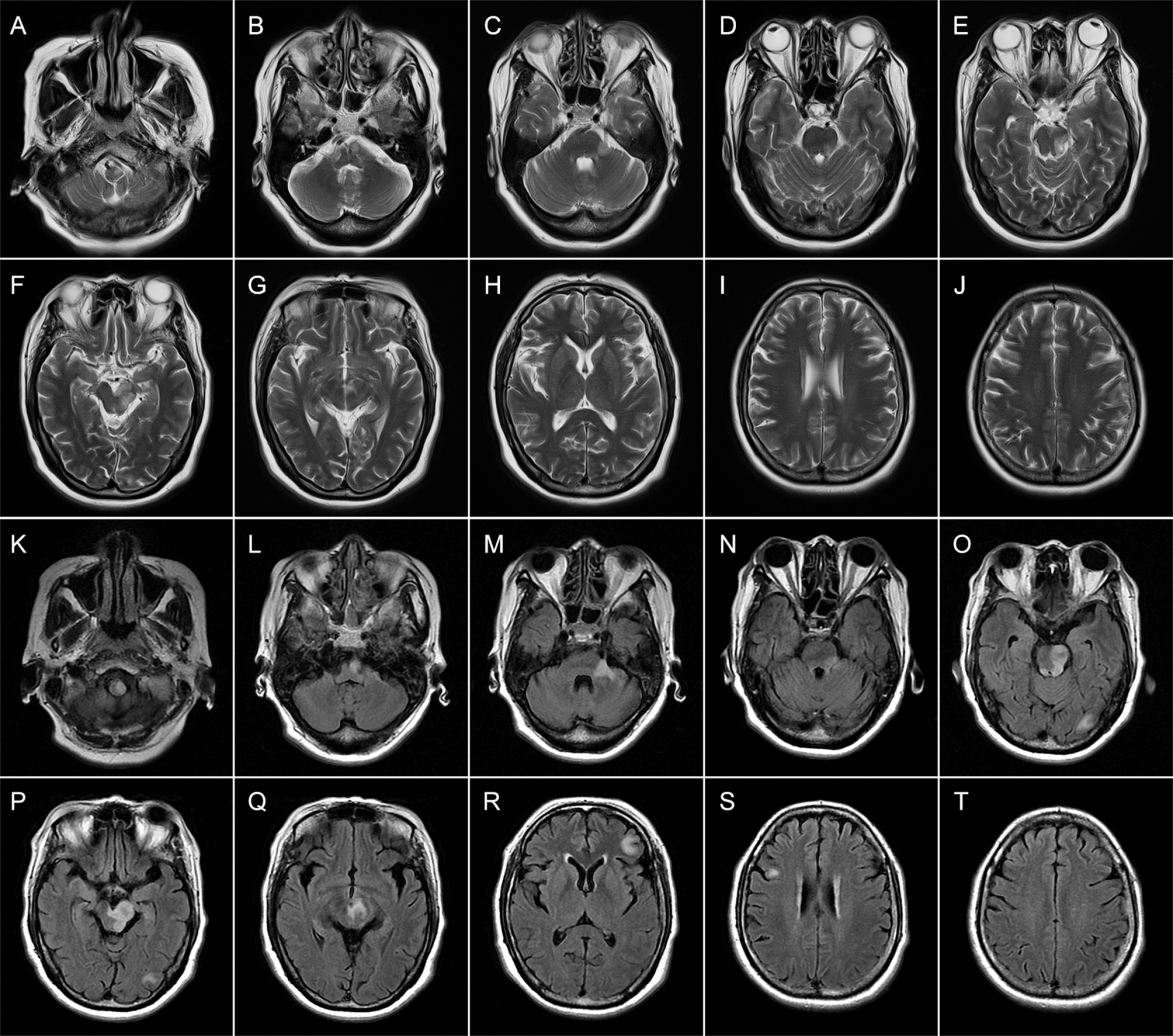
Brain magnetic resonance imaging (MRI) of patient 2 performed during acute attack. Axial T2-weighted (A–J) images obtained 4 days after onset show multiple patchy hyperintense lesions in the left brainstem (medulla, brachium pontis, and midbrain) (A–G) and juxtacortical white matters of the right frontal lobe (I). Axial FLAIR (K–T) images obtained 15 days after onset reveal expanding lesions in the brainstem (K–Q). Meanwhile, new patchy or spotty lesions are confirmed in the left occipital lobe (O, P) as well as juxtacortical and subcortical white matters of the left frontal lobes (R, T).
